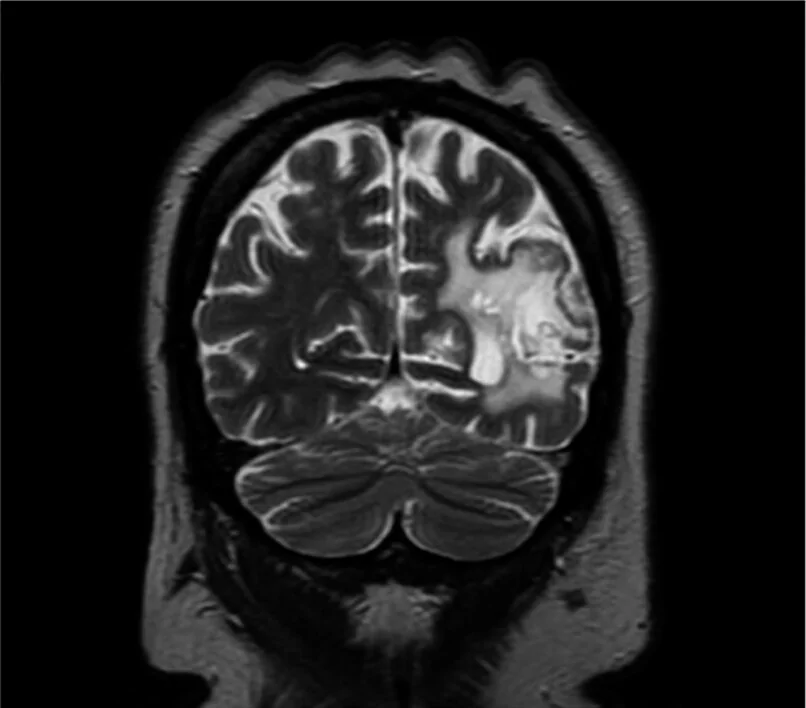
Magnetic resonance imaging (MRI) of the brain in Case 1 demonstrating chronic multifocal ischemic changes involving the left occipital, parietal, and temporal lobes, as well as bilateral frontal subcortical regions, without evidence of acute infarction.
